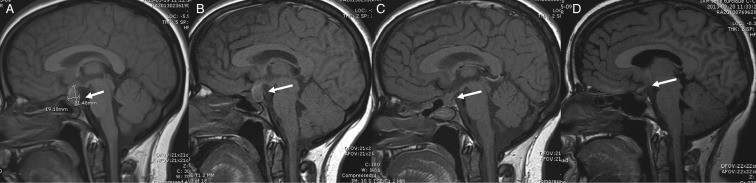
Cerebral MRI scans, sagittal T1. Arrows indicate the location of the mass. On admission and 1 week after, both pre-surgery (A and B): 1.7×1.1×1.9 cm rounded oval-shaped sellar lesion with suprasellar extension; immediately post-surgery (C) and 6 months post-surgery (D). We note the absence of the bright spot of the posterior pituitary on all four MRI scans. This can be predictive of a permanent central diabetes insipidus although specificity decreases with age.
